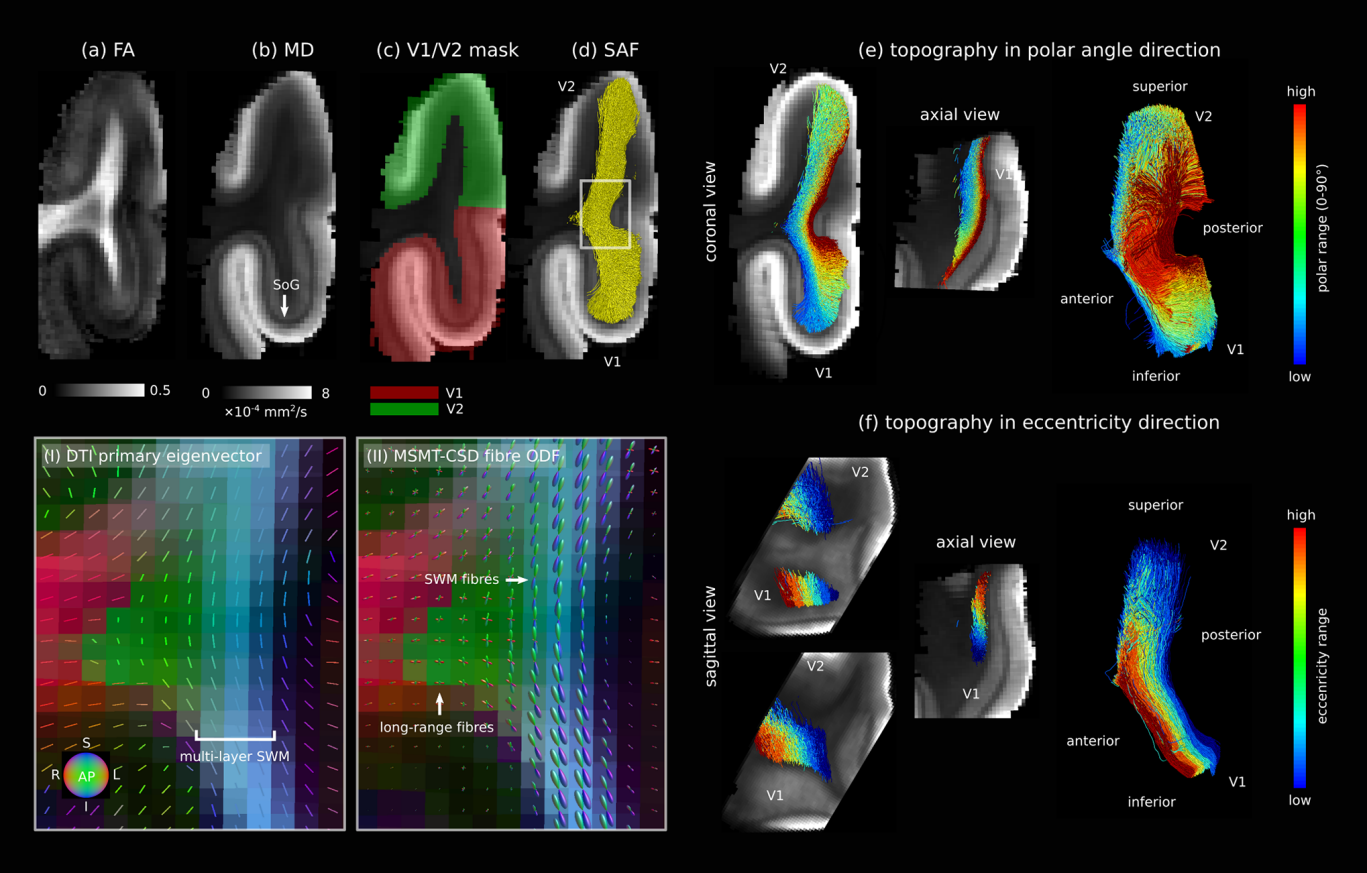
V1–V2 SAF geometry and topography mapped using 0.2 mm isotropic spatial resolution*post mortem*DWI. (a) Fractional anisotropy (FA) and (b) mean diffusivity (MD). Highly myelinated V1-specific Stria of Gennari (SoG) visible on MD. (c) Manually delineated V1 and V2 masks with SoG as landmark were used to map (d) the V1–V2 SAF. For the marked area in (d), dense and multi-layer superficial white matter fibres are shown in (I) DTI primary eigenvector and (II) multi-shell multi-tissue constrained spherical deconvolution (MSMT-CSD) fibre distribution maps. To demonstrate SAF topography, streamlines were clustered based on their location on the V1 cortical border using separate sets of manually created labels in the (e) polar angle and (f) eccentricity directions, giving different sets of output streamlines. V1–V2 SAF were topographically organised along both, as shown in planar and 3D views.
